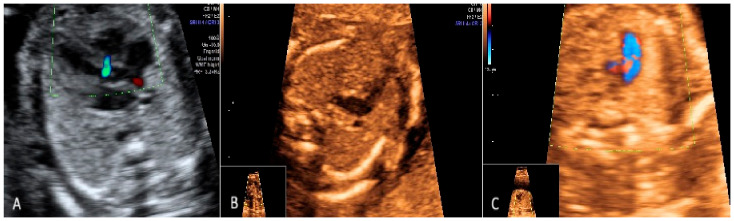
(A) Atrio-ventricular septal defect (Grey scale). (B) Transposition of the great arteries (Sepia). (C) Coarctation of aorta (Sepia -Color Doppler Mode).
